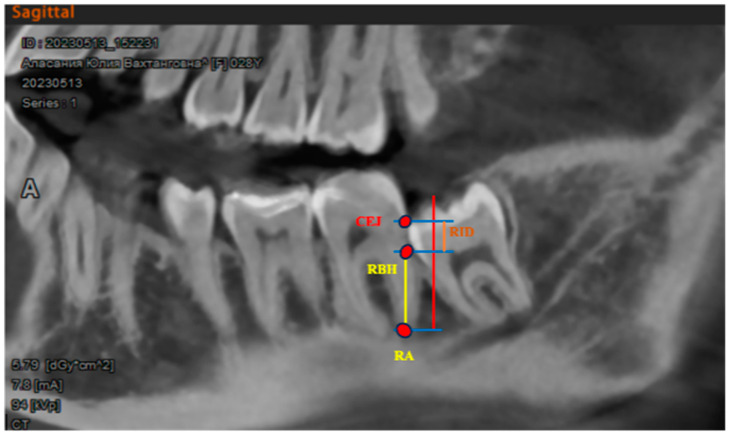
Radiology parameters: radiographic infrabony defect (RID); radiographic bone height (RBH); root apex (RA); cementoenamel junction (CEJ).
